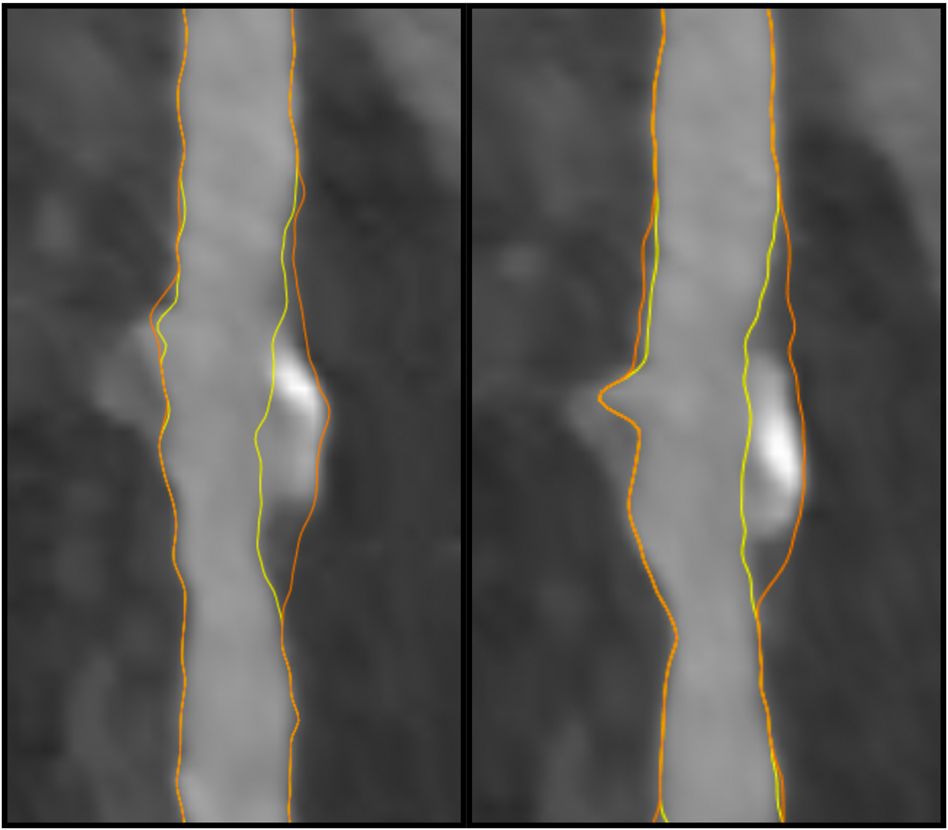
Example of coronary plaque. Left: Image from QAngio CT software of manually annotated CCTA examination showing a plaque in left anterior descending coronary artery. Yellow line, segmented vessel lumen. Orange line, outer contour of the vessel wall/outer wall of coronary plaque. Right: Fully automatic segmentation of the same examination by PlaqueViT (segmentation imported into QAngio CT software for visualization)
